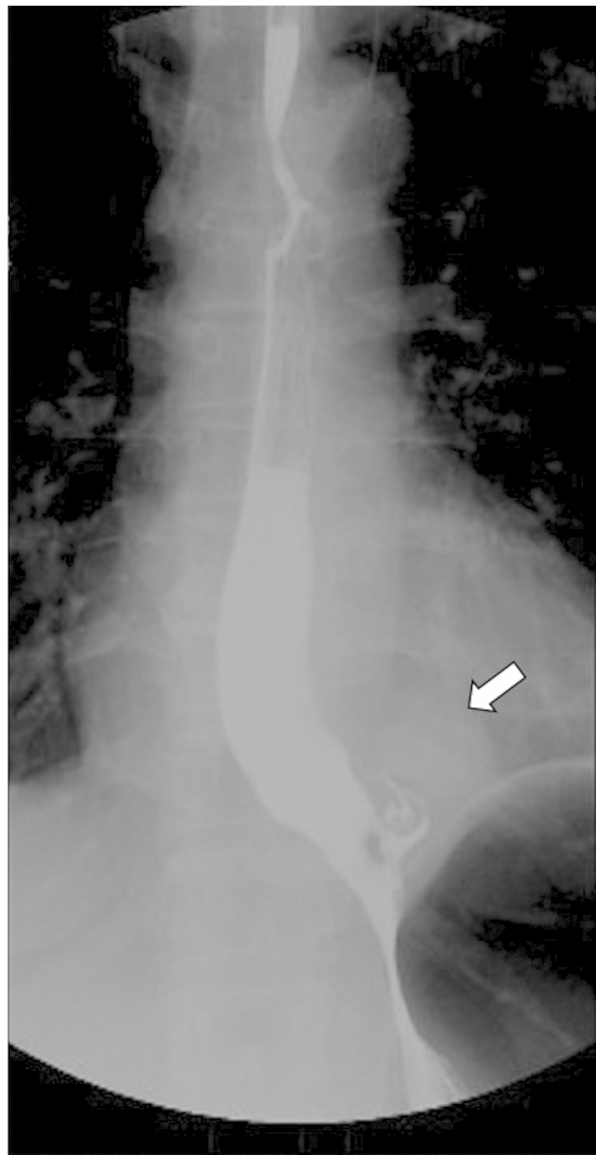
Upper gastrointestinal tract radiography revealed an esophageal submucosal tumor (arrow) at the distal esophagus.
